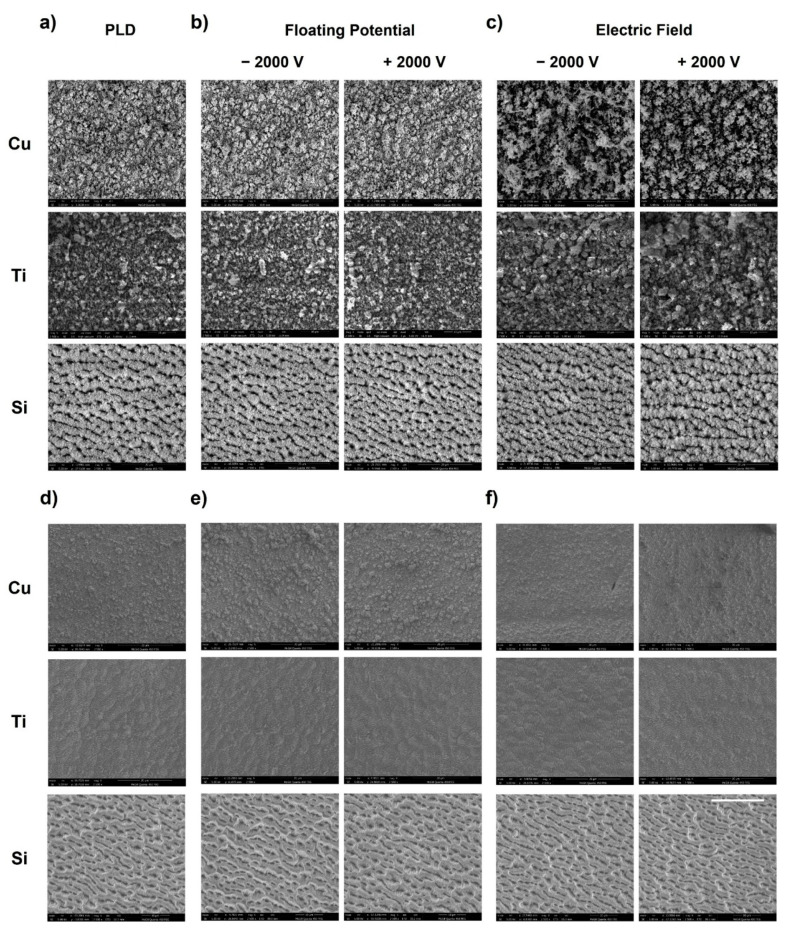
SEM images of Cu, Ti and Si surfaces laser micromachined in the parallel laser scanning direction under conventional PLD conditions (a,d), in the presence of a floating potential (b,e), and in the presence of an applied electric field (c,f), respectively, BEFORE and AFTER ultrasonication. The white scale bar corresponds to 20 µm and applies to all images.
